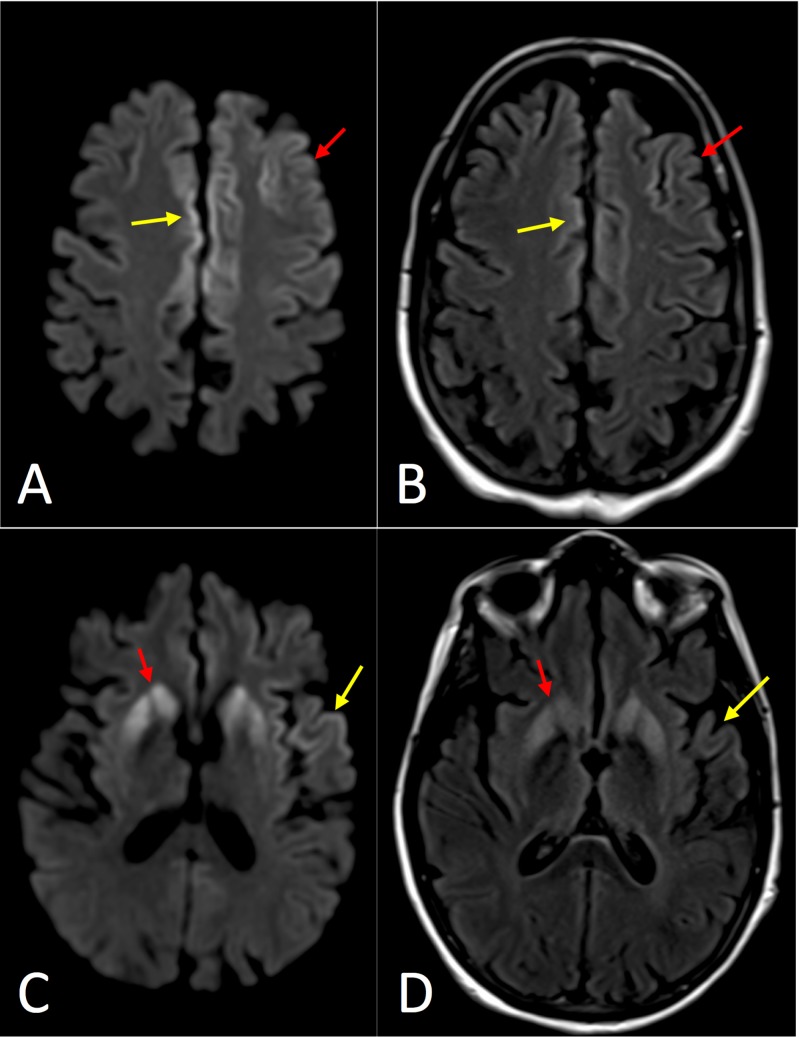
Axial magnetic resonance imaging (MRI) of Case 4. (A) Diffusion-weighted image (DWI) at the level of the centrum semiovale demonstrating asymmetrically increased signal in the frontal cortex (red arrow) on the left with notable involvement of the cingulate gyrus bilaterally (red arrow). (B) T2-weighted fluid attenuation inversion recovery (T2-FLAIR) image with less corresponding hyperintense signal. (C) DWI at the level of the basal ganglia demonstrating asymmetrically increased signal in the striatum (red arrow), left insular cortex, and left superior temporal gyrus (yellow arrow). (D) T2-FLAIR image with less corresponding hyperintense signal.
